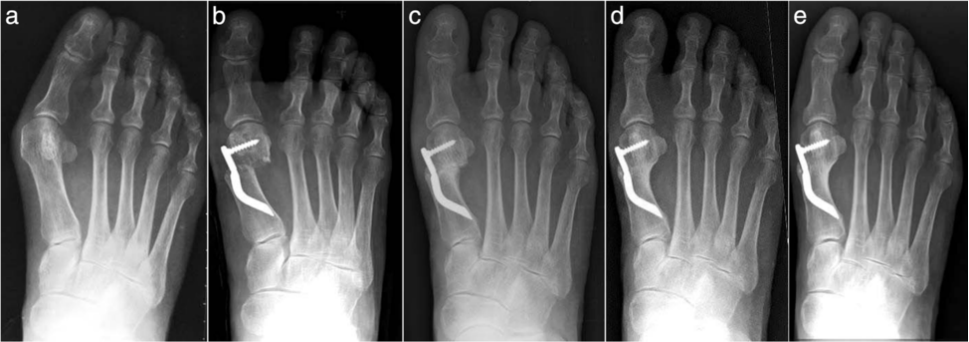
Case 9. A 71-year-old woman with moderate HV; antero-posterior radiographic images at: a Preoperative period. b 1-month follow-up. c 3-month follow-up. d 6-month follow-up. e 48-month follow-up showing the maintained correction of the deformity
